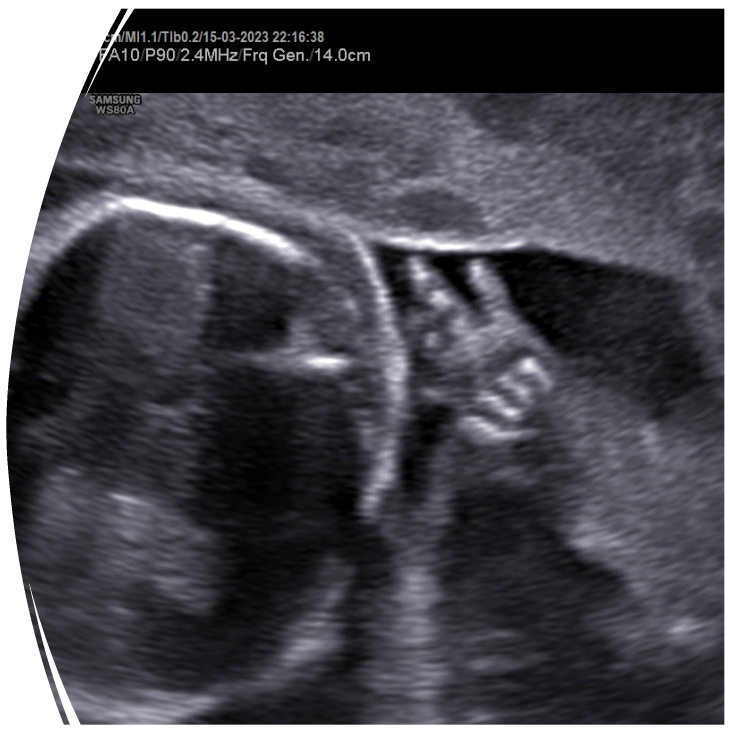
Βilateral clinodactyly was noted in the second-trimester ultrasound.
